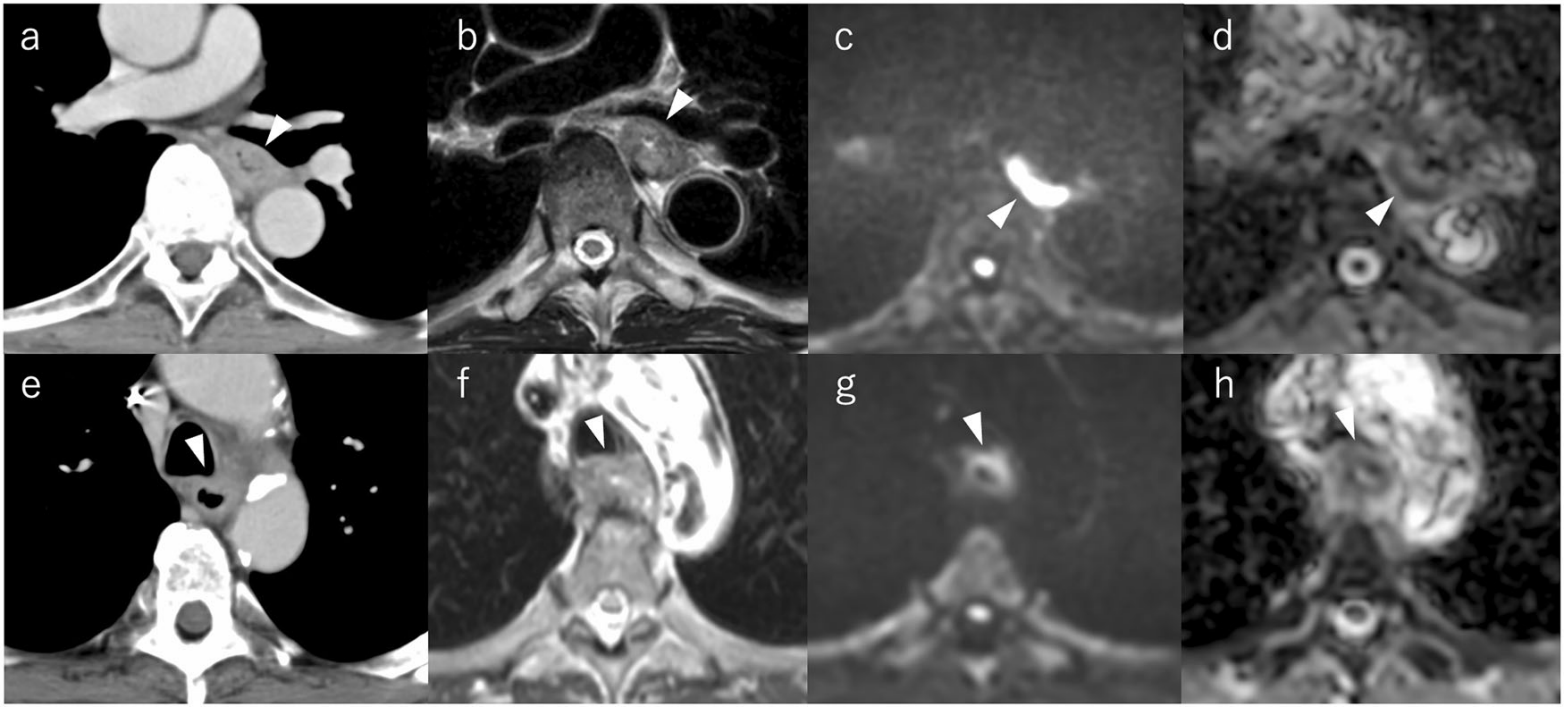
Representative cases of tracheal invasion. Upper row: The contrast-enhanced CT (a) shows compression of the left main bronchus and narrowing of the lumen due to the esophageal tumor (readers: cT4 vs. cT3br), whereas in the MRI T2WI (b), although the esophageal muscular layer is partly unclear, the low-density line of the left main bronchus is maintained, and in DWI (c) and ADCmap (d), the localized decrease in diffusion is limited to the posterior wall of the esophagus, and it was judged that there was no obvious infiltration (both readers: T-score 3). Lower row: Contrast-enhanced CT (e) suggests slight bronchial irregularity (readers: cT3br vs. cT3r), but MRI T2WI (f) and DWI (g), ADCmap (h) demonstrate loss of the bronchial wall’s low-intensity line (both readers: T-score 5). Pathology confirmed pT4
